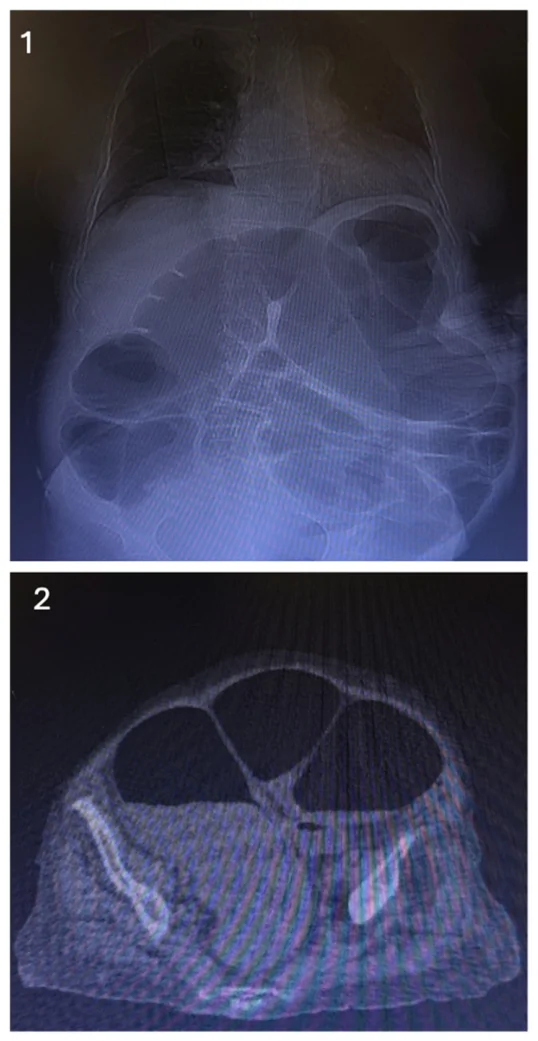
Preoperative imaging of a patient diagnosed with sigmoid volvulus. (1) Erect plain abdominal radiograph: a massively dilated, air-filled sigmoid colon segment, consistent with the classic “coffee bean” appearance of sigmoid volvulus. (2) Axial computed tomography slice: the same markedly dilated bowel segment and thinned wall structure are seen. These imaging findings directly correspond to the intraoperative exteriorization view shown in Figure 5.
